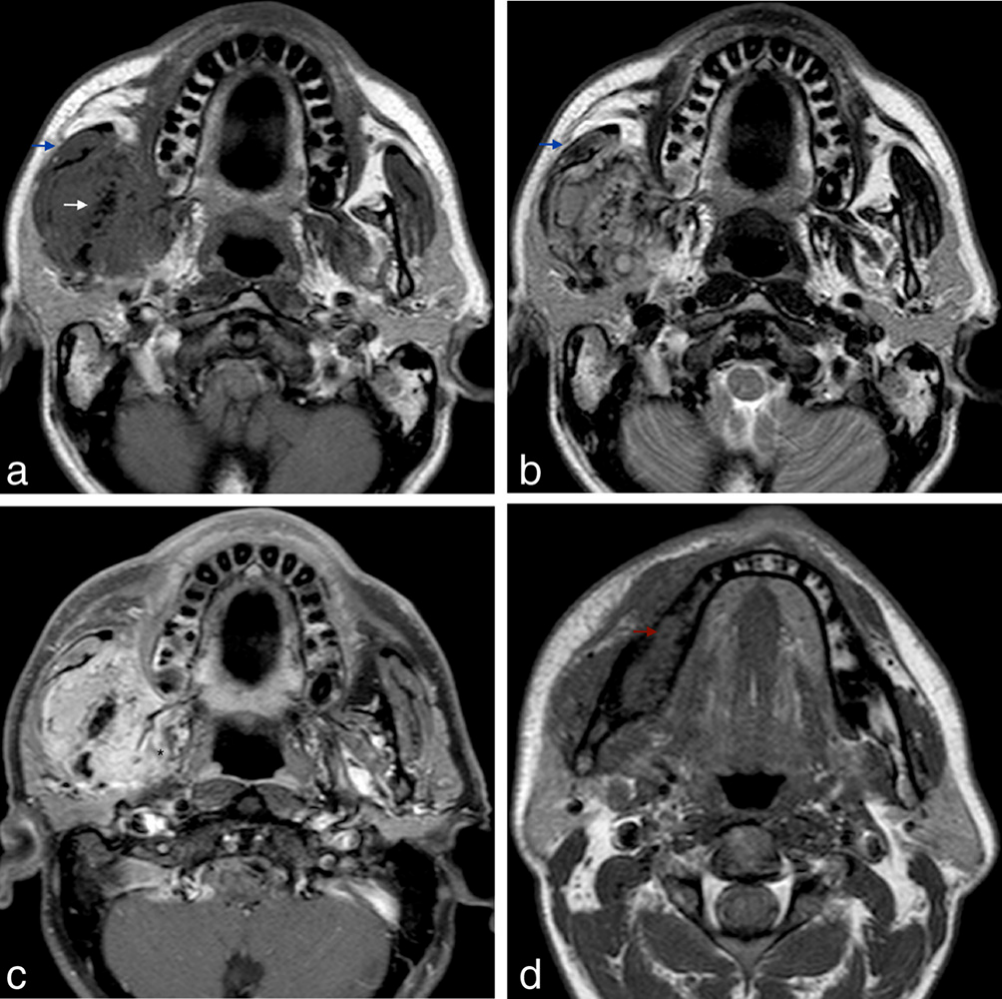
(a, b) Synchronized axial *T*_1_ weighted and *T*_2_ weighted MR images show multiple coalescent hypointense foci in the region of the mandibular ramus (white arrow) and a surrounding soft tissue component isointense and hyperintense in relation to the remaining masseter (blue arrows) on *T*_1_ weighted image and *T*_2_ weighted image, respectively. (c) Axial enhanced fat-suppressed *T*_1_ weighted MR image demonstrates avid tumour enhancement and better depicts the involvement of the pterygoid space (asterisk). (d) Axial *T*_1_ weighted MR image at a lower level shows the replacement of the normal fatty marrow by an expansive soft tissue mass expanding and remodelling the cortical bone (red arrow).
